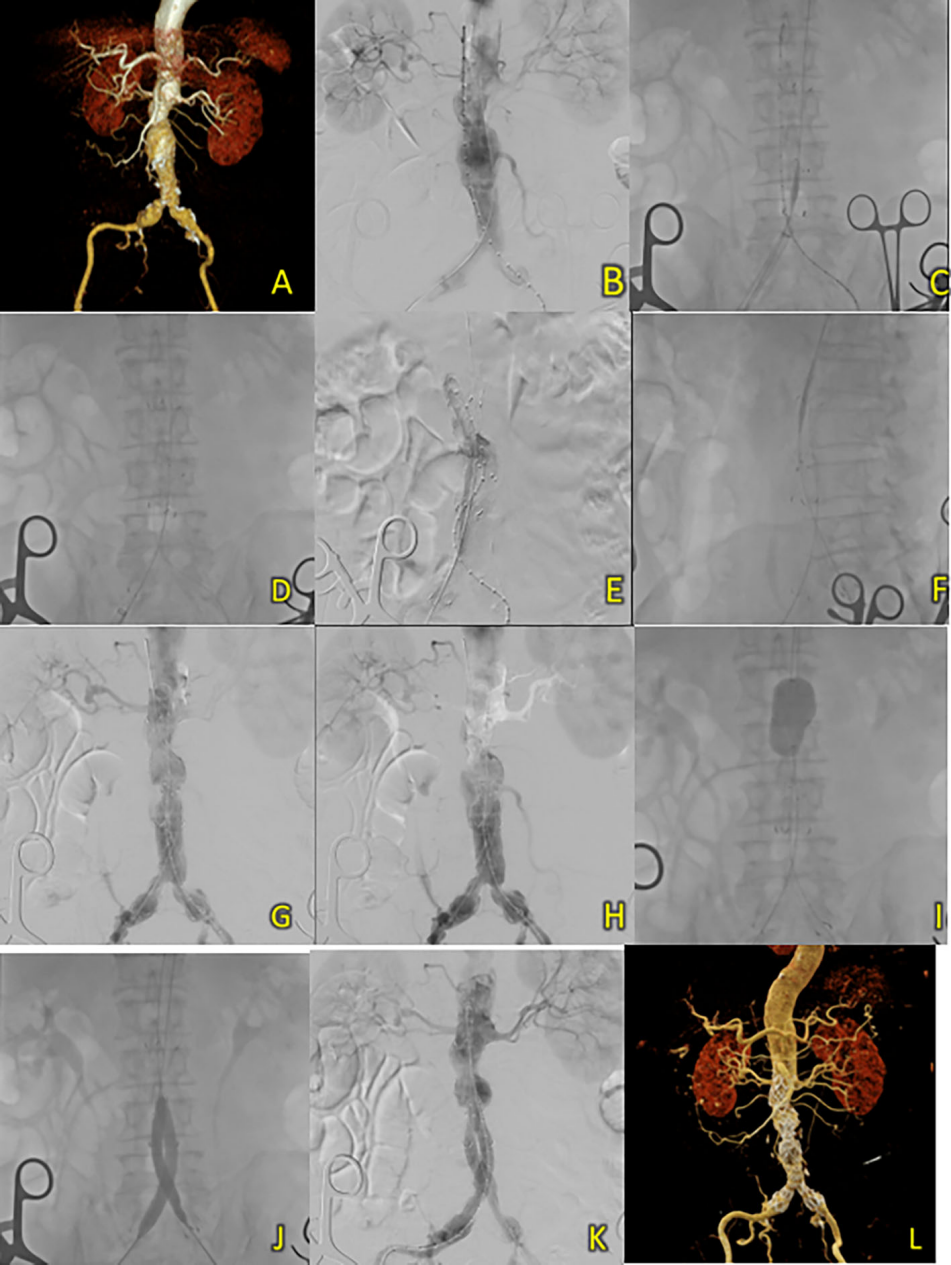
T&K B-EVAR on Patient 6. (A) Pre-procedure CTA. (B) Angiography reveals an infrarenal fusiform aneurysm. (C-F) T&K B-EVAR step-by-step. (G-H) Underexpansion of graft stent. (I-J) Balloon dilatation of main body graft and limb extensions. (K) Evaluation angiography reveals good graft placement and no endoleak. (L) Post-procedure CTA shows good results.
